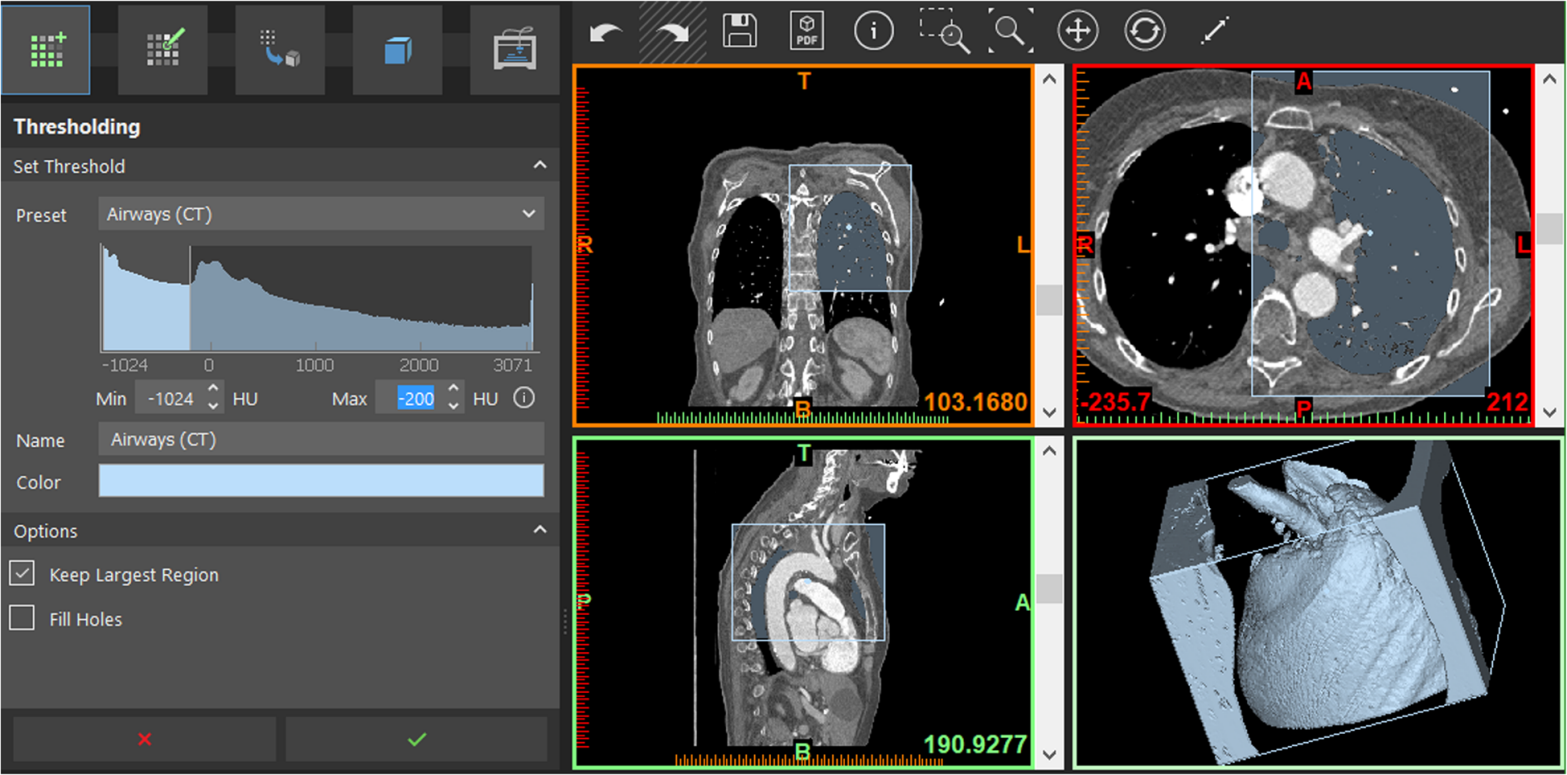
Segmenting the airways using the Airways (CT) preset
